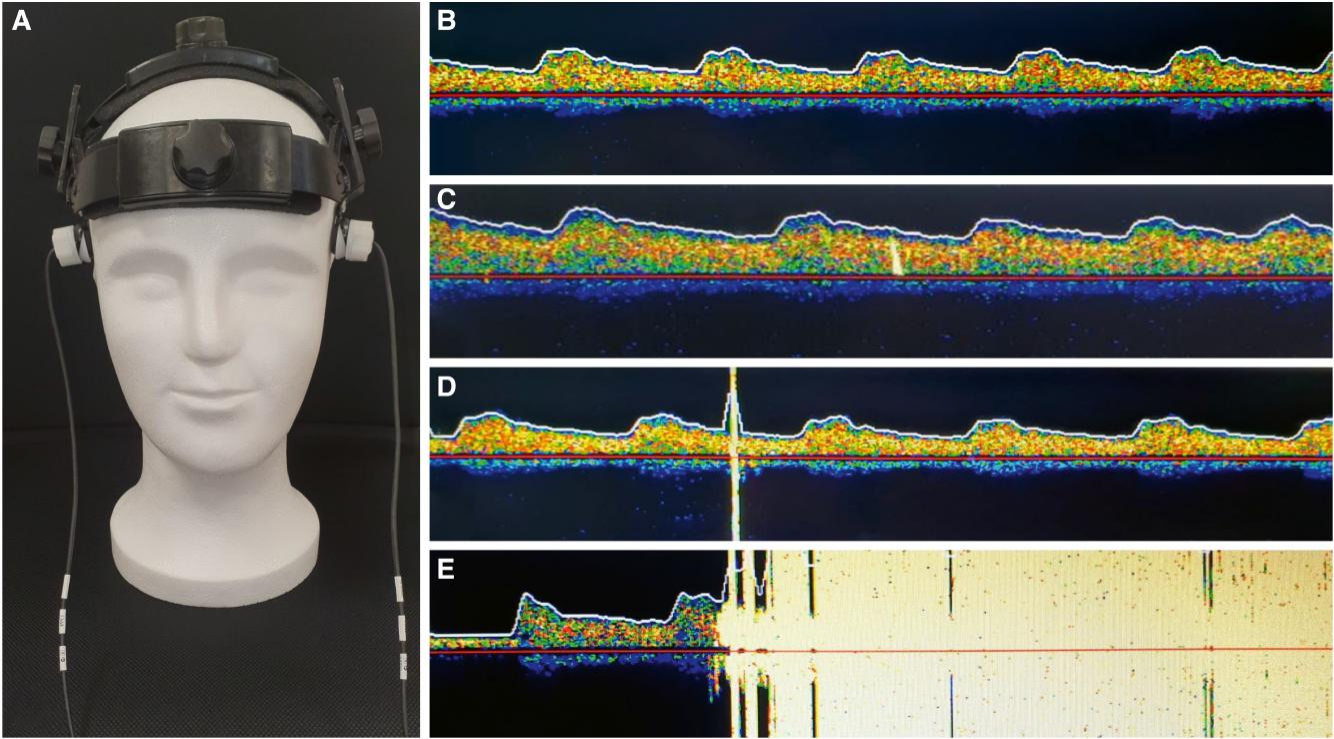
Microembolic signal detection using Transcranial Doppler Ultrasonography. (*A*) Transcranial Doppler ultrasonography displaying the flow signal over the arteria cerebri media; (*B*) normal flow profile over the middle cerebral artery; (*C*) high-intensity signal (HITS) with strictly unidirectional signal within the Doppler spectrum implying a ‘solid’ embolus; (*D*) large, high-intensity bidirectional signal (HITS) exceeding the Doppler spectrum implying a ‘gaseous’ embolus; (*E*) many (innumerable) HITS, indicating a shower. *Adapted and modified from Erkapic et al.*^11^ HITS, high-intensity signal.
